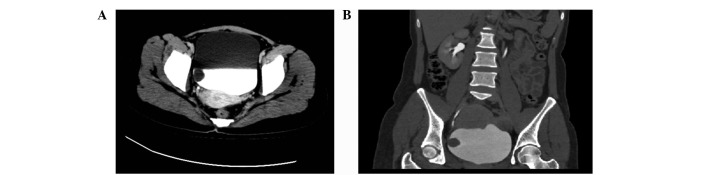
Axial and coronal views from computed tomography scan revealing the position of the tumor protruding into the bladder with an intact surface.
